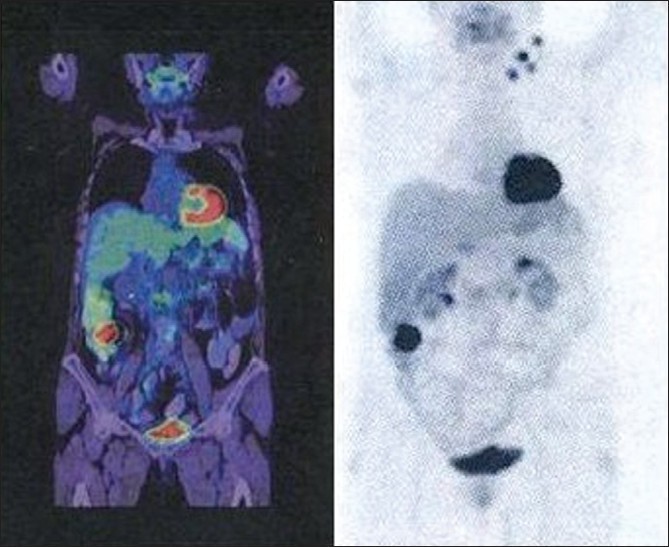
Fluorodeoxyglucose-Positron Emission Tomography. Increased uptake in the correspondence of the right colon (Standardized Uptake Value, SUV = 12.1)
